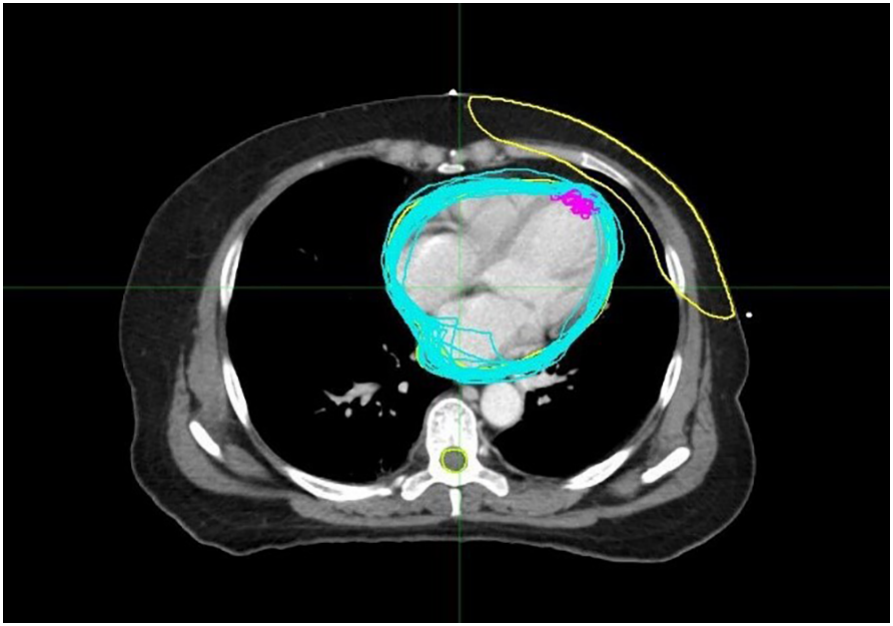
The *blue lines* represent the outlines of the heart traced in each cone beam CT (CBCT) image, while the *purple lines* represent the outlines of the left anterior descending coronary artery (LAD) traced in each CBCT.
